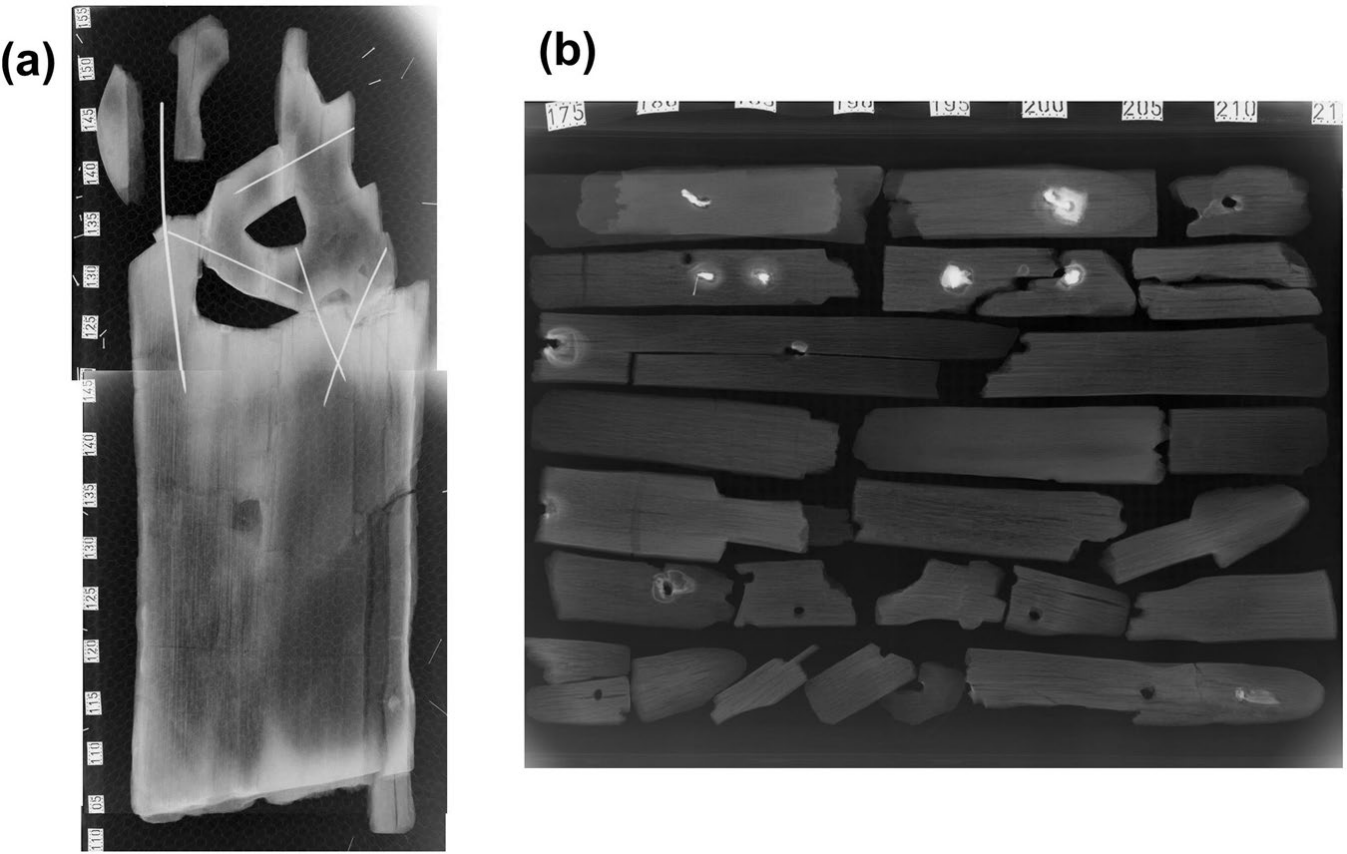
X-ray images of (a) object 250, showing metal rods (bright white lines) from reconstruction; (b) fragments of object 210, some with remnant nails, seen as bright white areas in the image.
